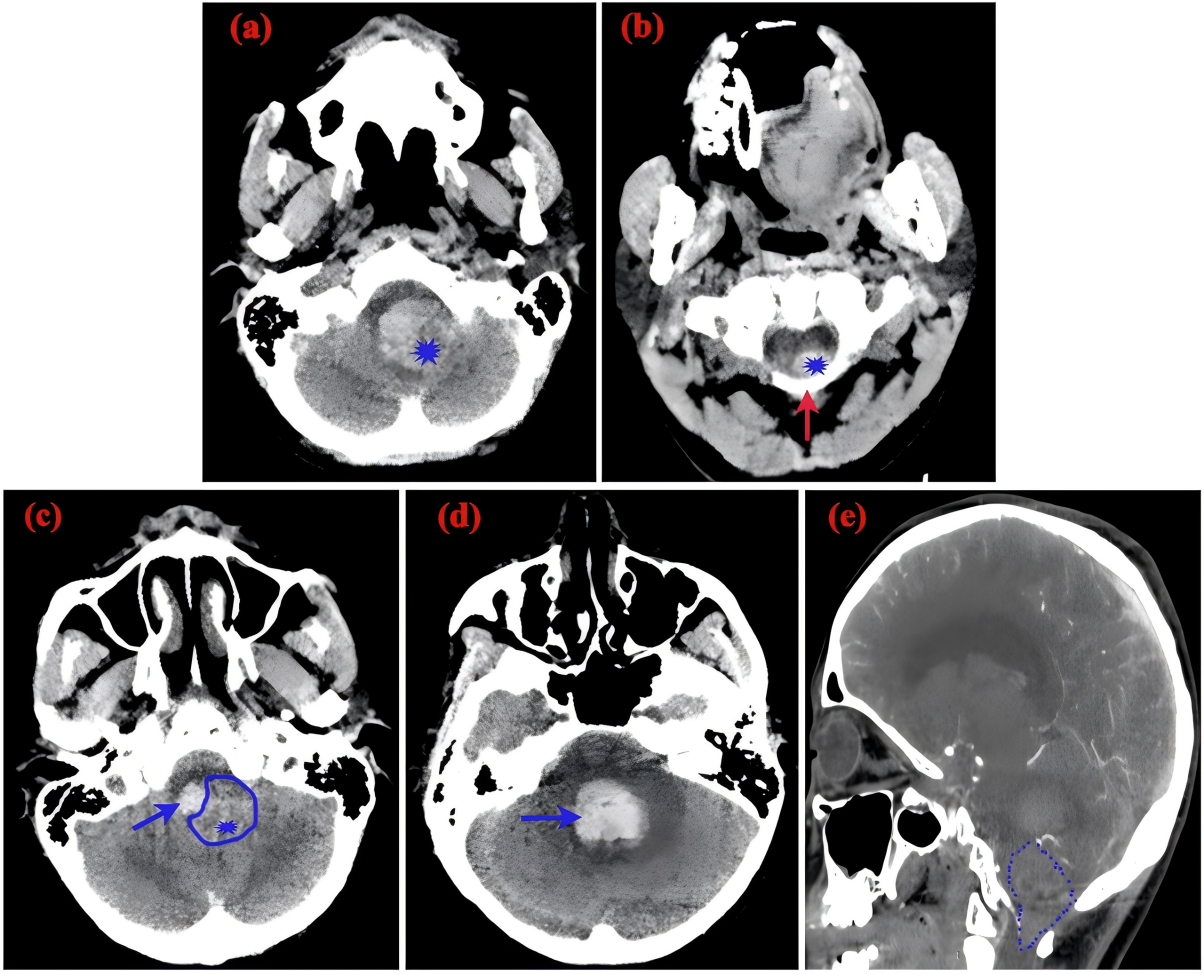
CT scans(a) Slightly hyperdense mass in the cerebellar vermis, indicated by the blue asterisk (*), accompanied by a hyperdense hemorrhagic lesion on the right. (b) Slightly hyperdense mass extending inferiorly to the cervical spinal cord at the C1 segment (blue asterisk); the red arrow denotes the lamina of the atlas (C1). (c) Slightly hyperdense mass in the cerebellar vermis (blue asterisk) and hemorrhage within the fourth ventricle (blue arrow). (d) Blue arrow denotes hemorrhage within the fourth ventricle. (e) Sagittal view of original CTA image demonstrating the size and location of the tumor (highlighted in blue).
